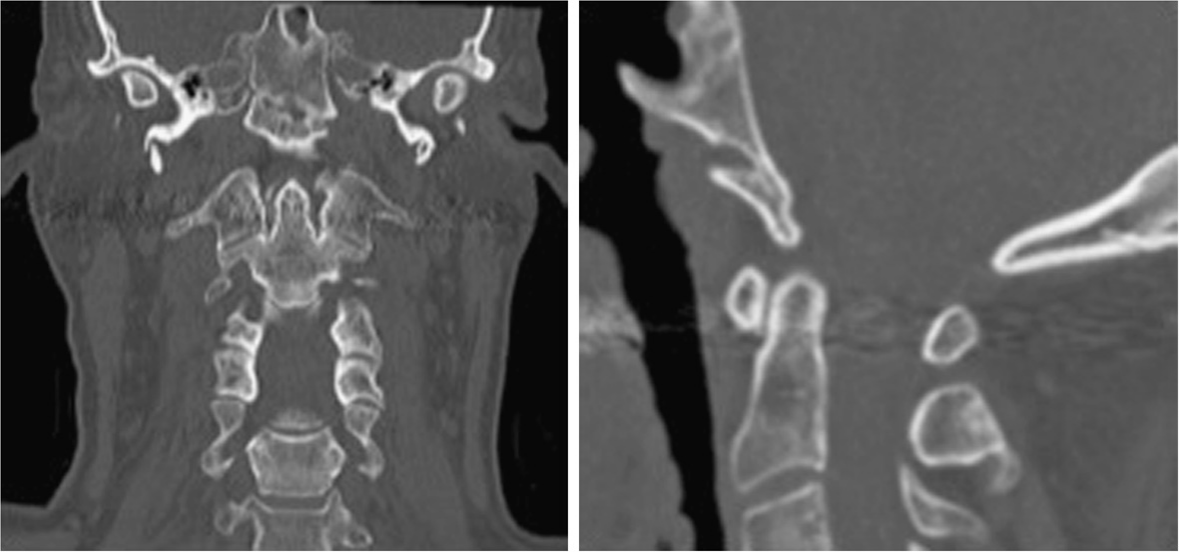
Computed tomography of the cervical spine after 12 weeks of immobilization with a halo-device, proving the consolidation of the fracture.
